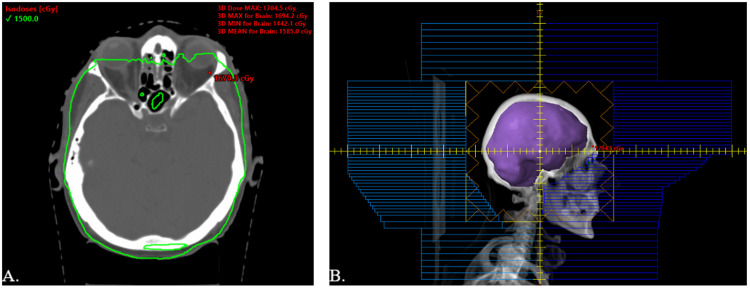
Radiation treatment plan. (A) Radiation treatment plan of 15 Gy in five fractions using 6-MV opposed lateral photon beams. (B) Digitally reconstructed radiograph of the treatment field with an inferior border at C2-C3.
